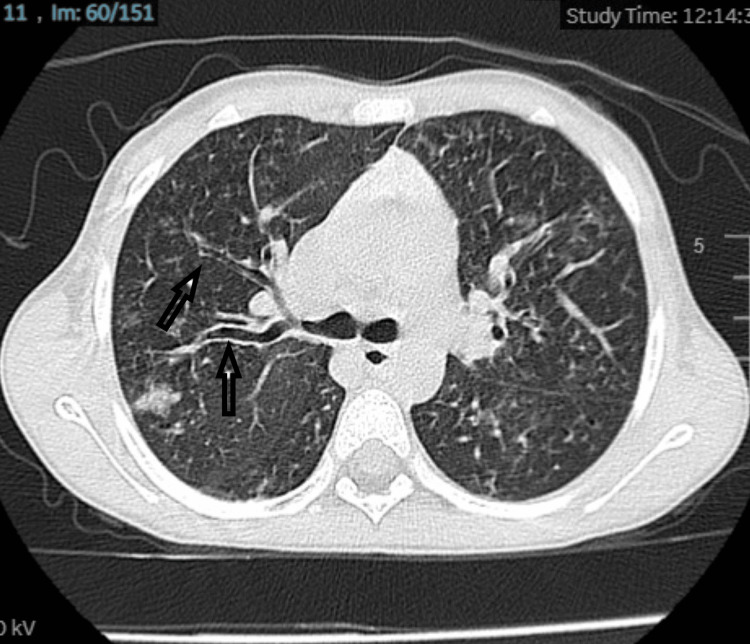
CT image of the chest CT chest revealed a tree in the bud with tubular bronchiectasis (black arrow).
